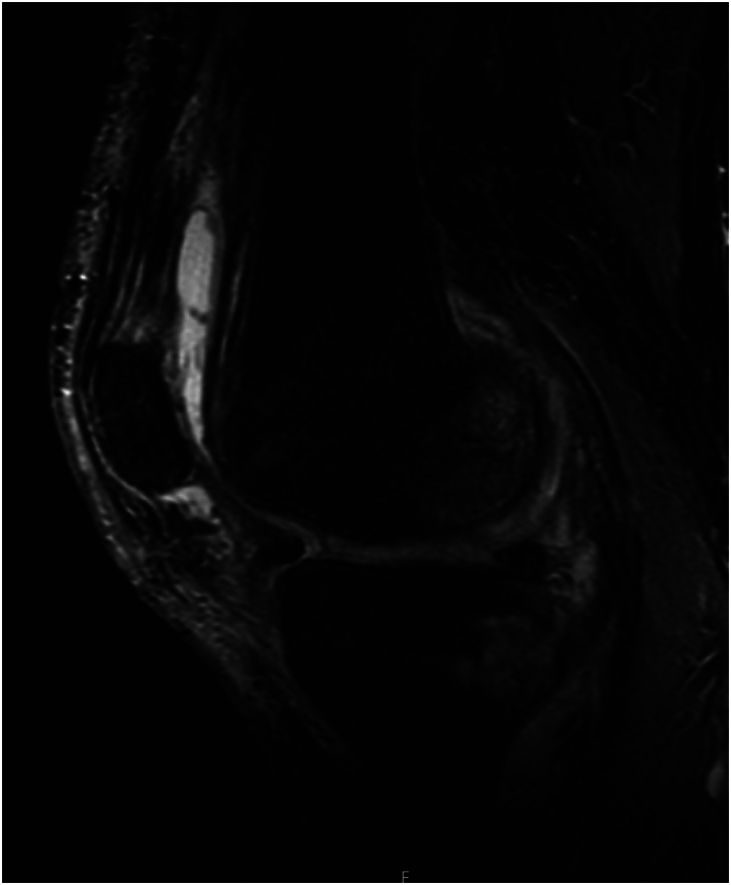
Preoperative MRI showing native posterior tibia and femur osteomyelitis with recurrent effusions, pain, and elevated inflammatory markers. MRI, magnetic resonance imaging.
